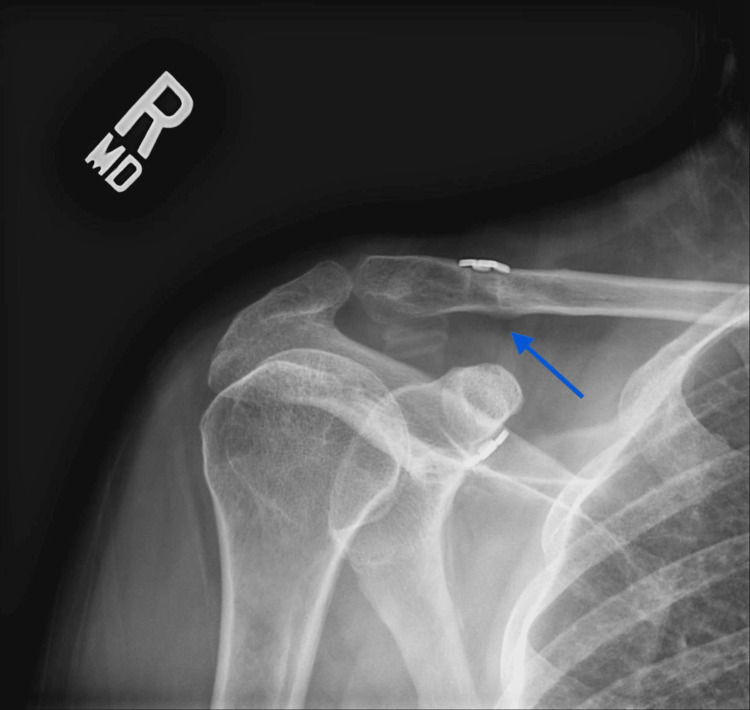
Twelve-month postoperative radiograph demonstrates significant tunnel widening (blue arrow) in the clavicle
